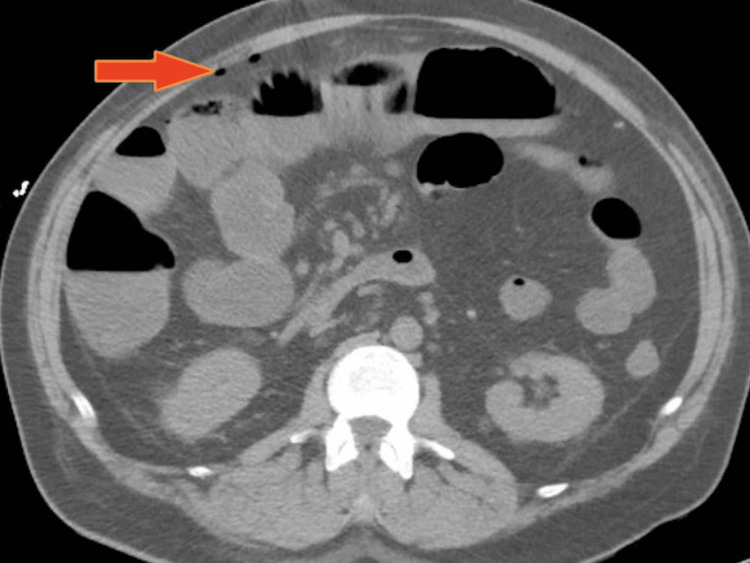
CT abdomen showing extra-intestinal foci of air suggestive of bowel perforation. The red arrow shows extra-intestinal foci of air.
